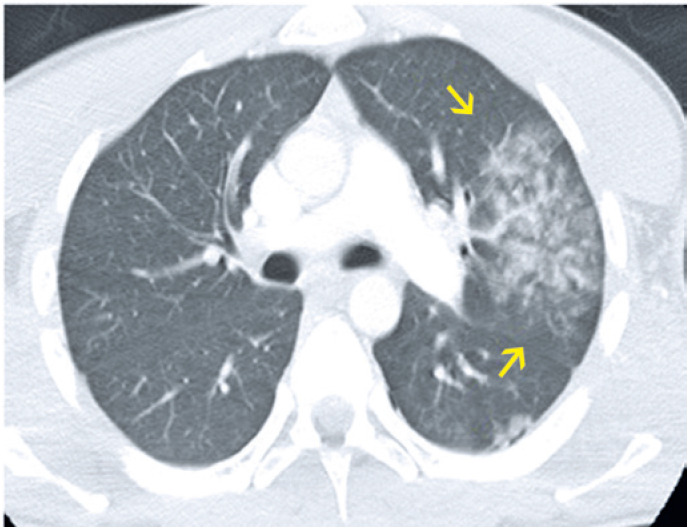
A 19-year-old man had been in a motorcycle accident. Computed tomography shows foci of consolidations located in the upper left lobe and, to a lesser extent, in the lower lobe on the same side. These findings are compatible with pulmonary contusions within the clinical context
